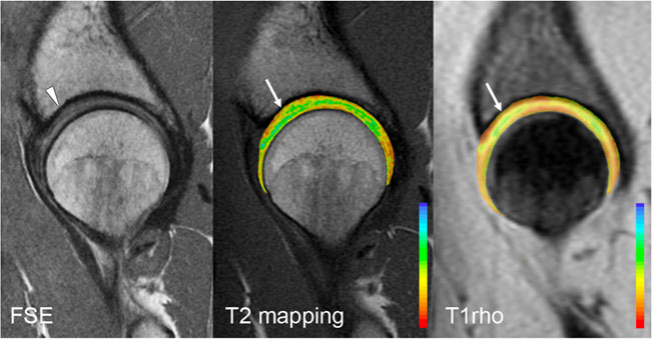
Sagittal PD-weighted MRI of the hip in a 30-year-old woman demonstrates mild chondral hyperintensity over the anterosuperior acetabular dome (*arrowhead*), with corresponding prolongation of relaxation times on T2 mapping and T1rho images (*white arrows*)
